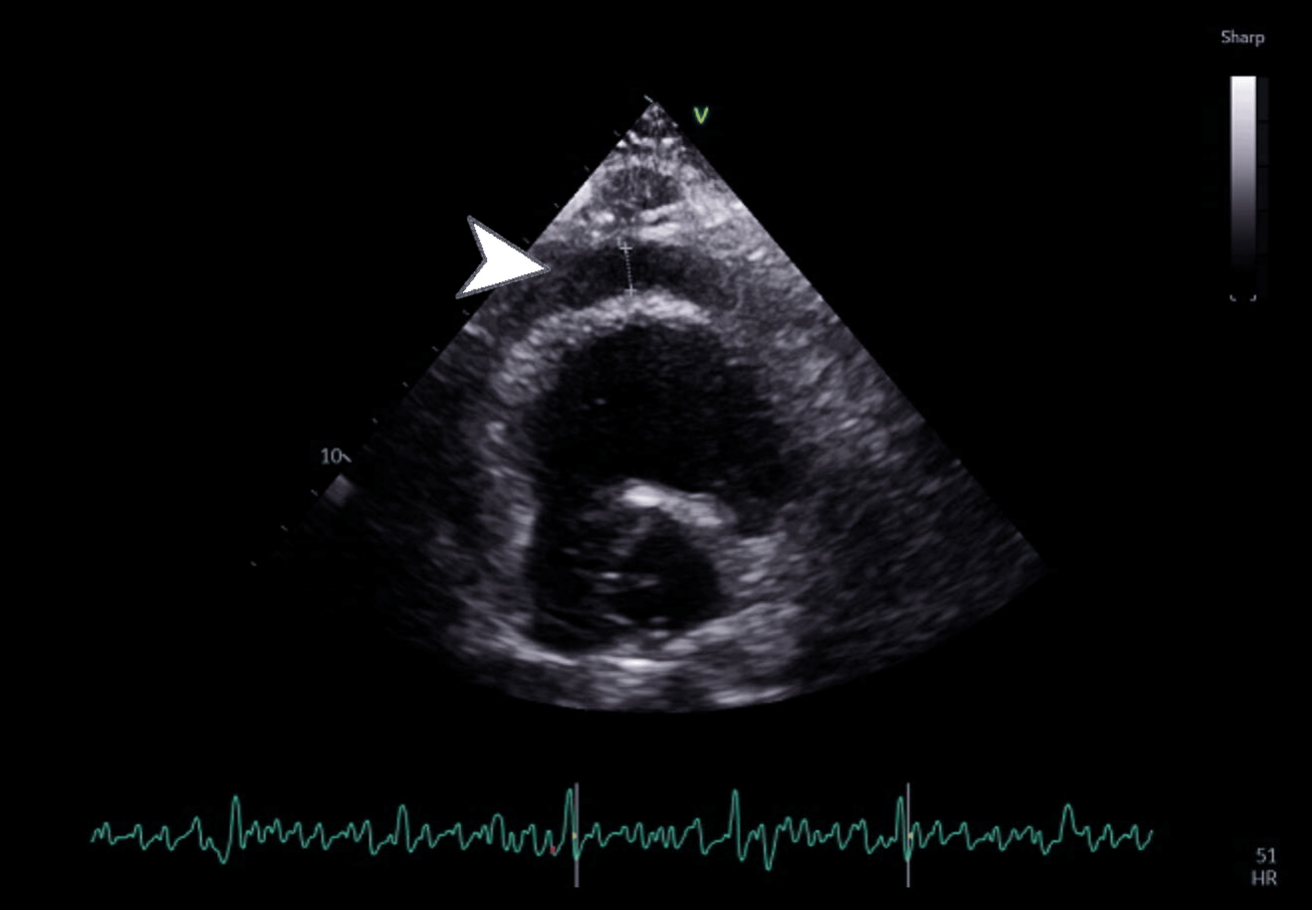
Echocardiogram showing pericardial effusion next to the right ventricular outflow tract (still frame)
